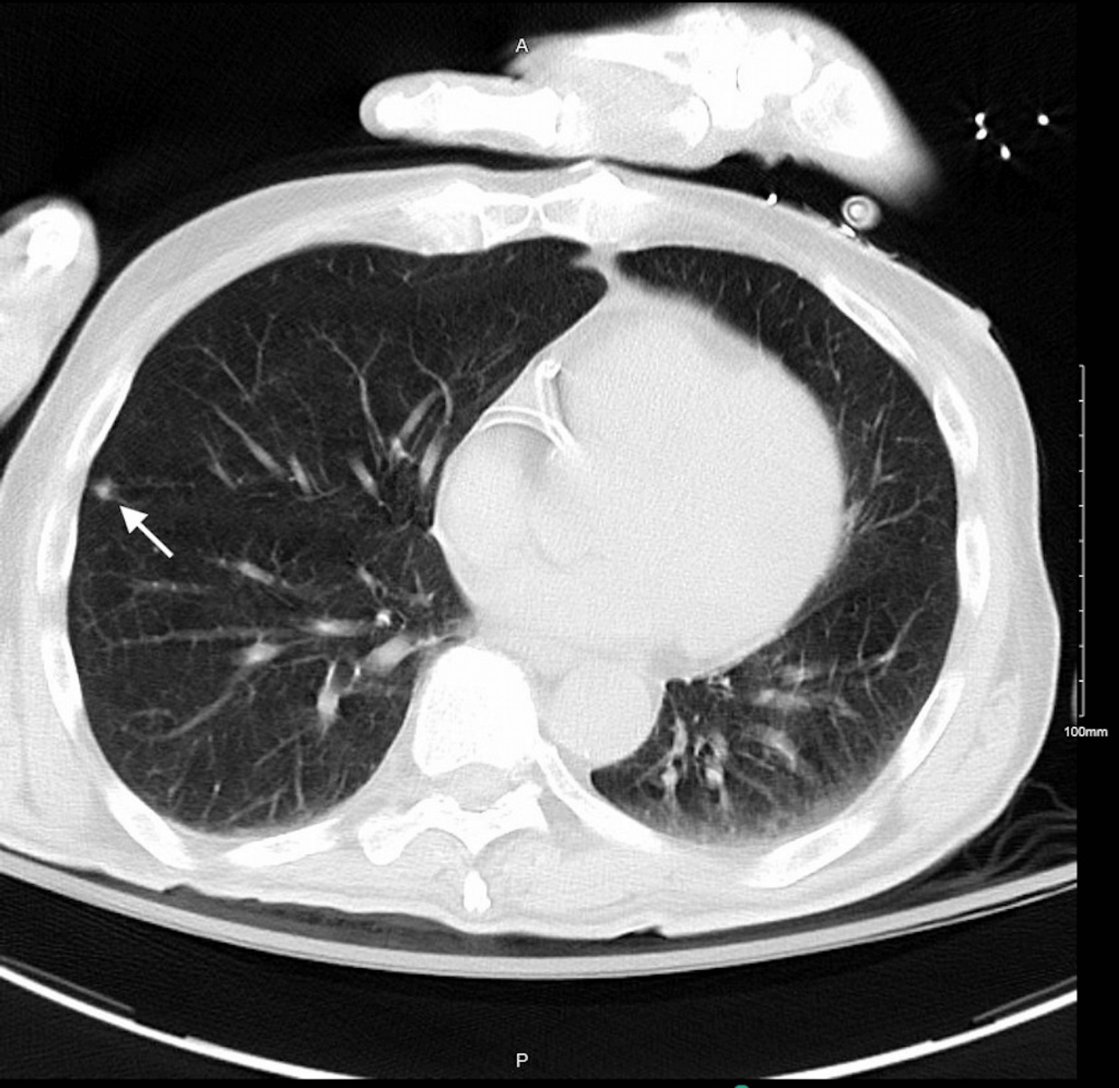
Computed tomography of the chest showing a 6 mm nodule (arrow)
